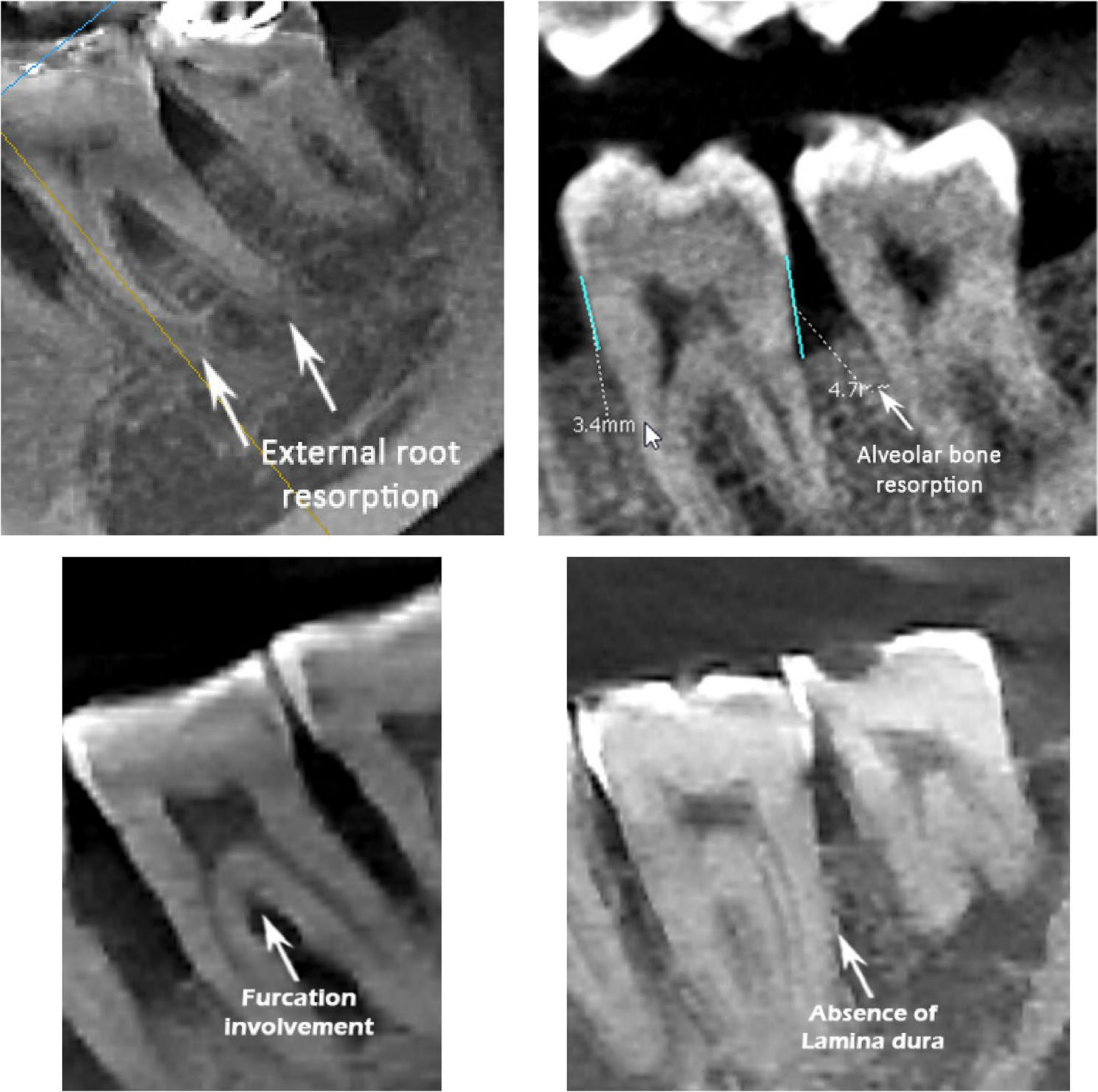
CBCT sagittal sections showing M2’s dental caries, ERR, ABR, FI and the absence of LDs
